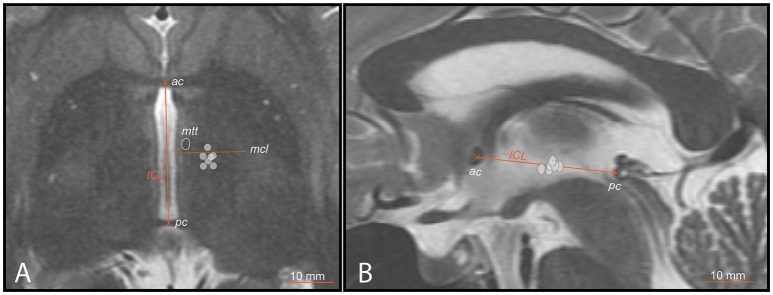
PTT target lesion sub-units (ICL ≥26mm) (gray circles) on preoperative MR images. (A) axial scan centered on the intercommissural plane (DV0). ICL is the distance between the center of the anterior (ac) and posterior (pc) commissures. The mid-commissural line (mcl) is represented as dotted line. (B) mid-sagittal scan onto which target lesion sub-units 1 to 7 (ICL ≥26mm) are projected.
